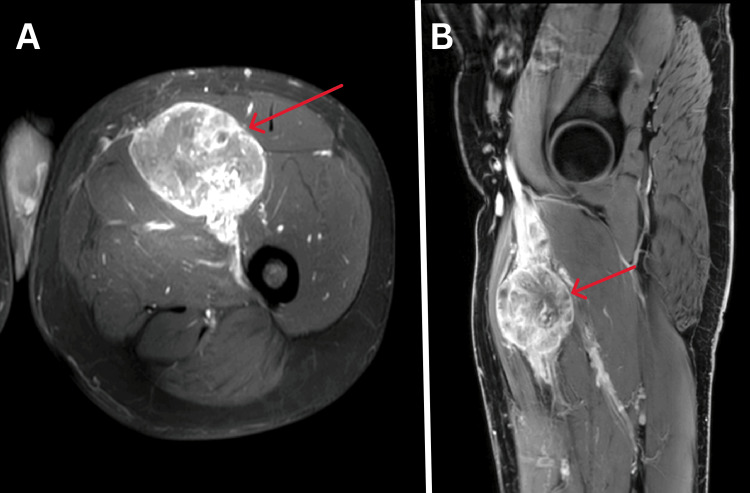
Contrast-enhanced magnetic resonance imaging (MRI) of femoral vein leiomyosarcoma. A) Axial and B) Sagittal T1 weighted MRI images show well-defined heterogeneously enhancing solid cystic lesions along with femoral vein (red arrow) and intravenous extension.
